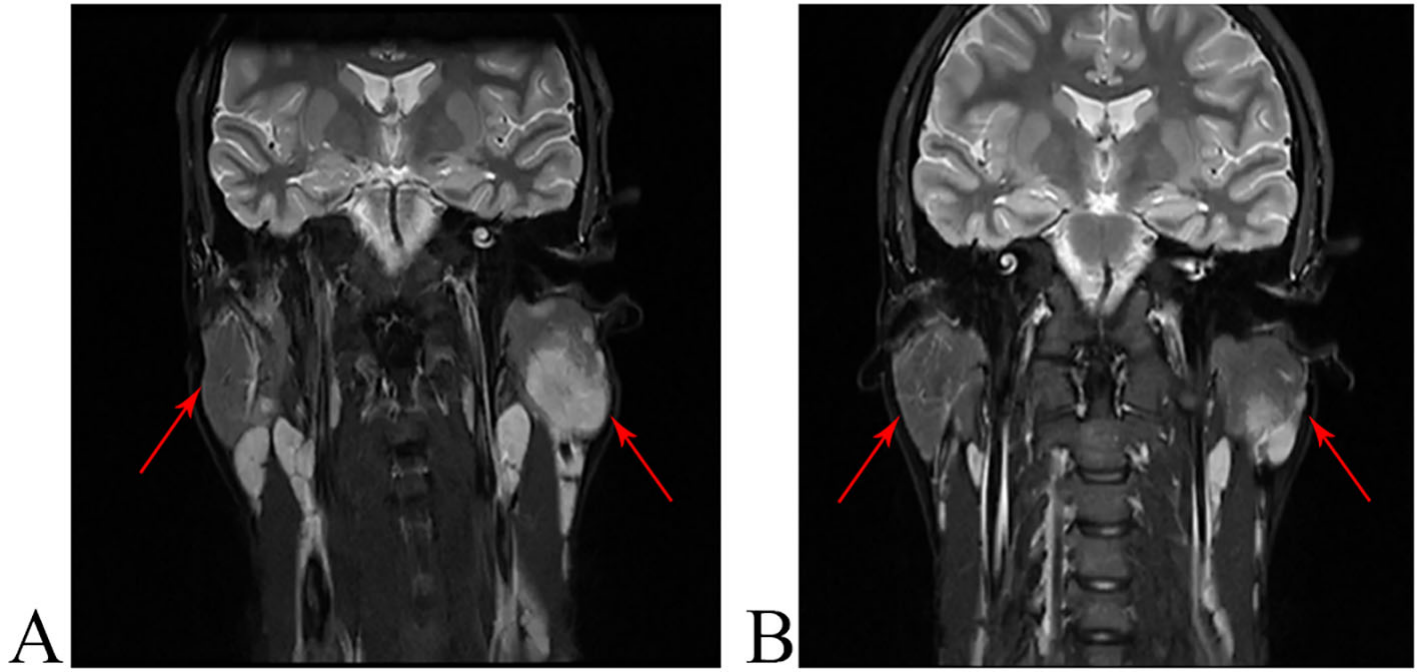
Radiological appearance of KD lesions on short-tau inversion-recovery T2-weighted fast spin-echo images. (A) The MRI results before treatment with dupilumab showed multiple space-occupying lesions in both parotid glands, with the largest measuring 2.8×2.3×3.5cm. (B) At week 20 of treatment, the MRI still showed multiple space-occupying lesions in both parotid glands, but the largest one measured only 1.8 × 0.6 × 2.4 cm.
